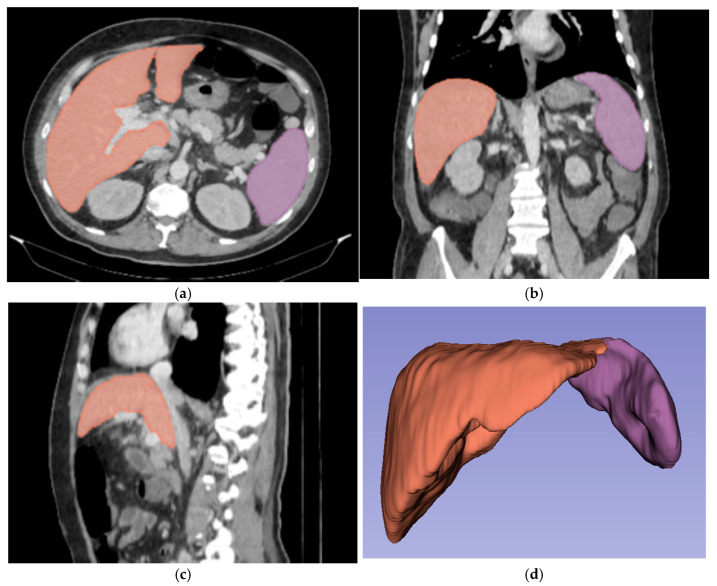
Abdominal portal-venous-phase CT scan: axial, coronal, and sagittal planes (a–c). Example of 3D liver and spleen segmentations. Three-dimensional liver and spleen segmentations were performed by using the TotalSegmentator extension in Slicer software, followed by manual corrections. Images (a–c) show the liver and spleen segmentation in axial, coronal, and sagittal planes. Image (d) shows the final 3D segmentation of these organs.
